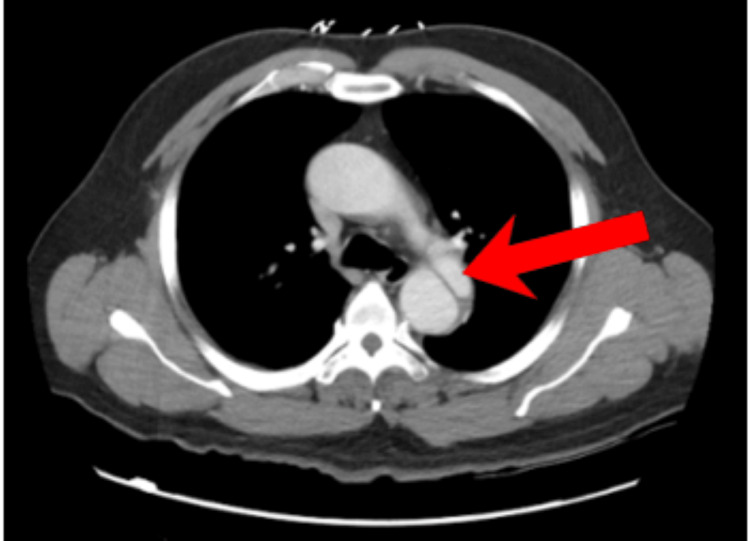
CT chest axial view of aortic dissection (false lumen in descending aorta, red arrow) CT: computed tomography
